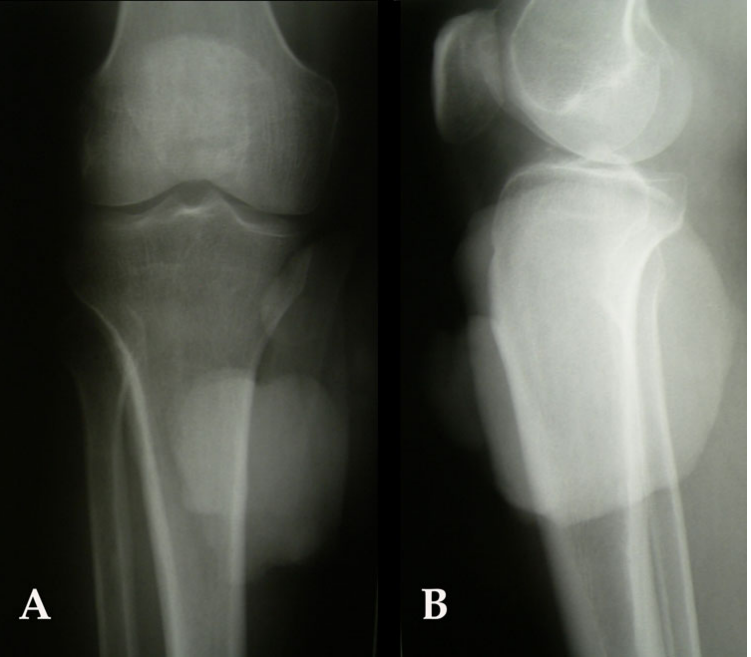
Anteroposterior (A) and Lateral (B) X-Ray view of the right knee. A soft tissue mass in the anterior-medial side of the proximal tibia with no evident continuity with the bone or cortical invasion was apparent.
